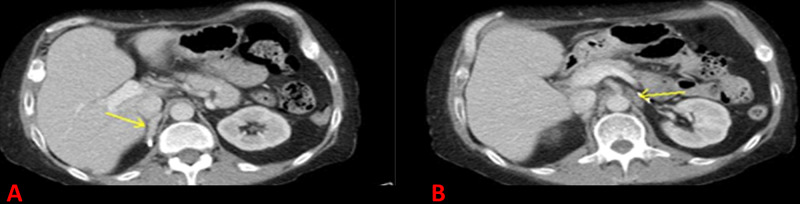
Computed tomography scan of the abdomen: (A) right adrenal gland (arrow), and surgical clip from previous adrenalectomy is seen posterior to the gland. (B) left adrenal gland (arrow).
